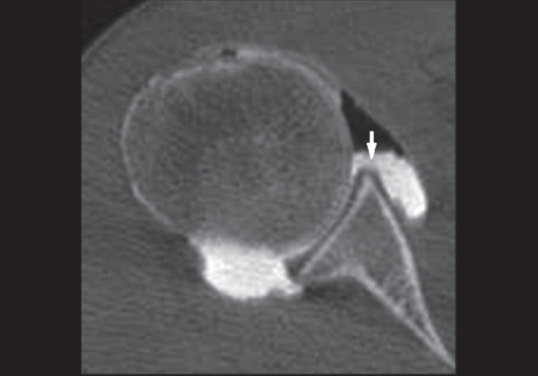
Axial CT-Arthrography image of a 33-year-old man with arthroscopy proved absence of the antero-inferior labrum (*arrow*)
